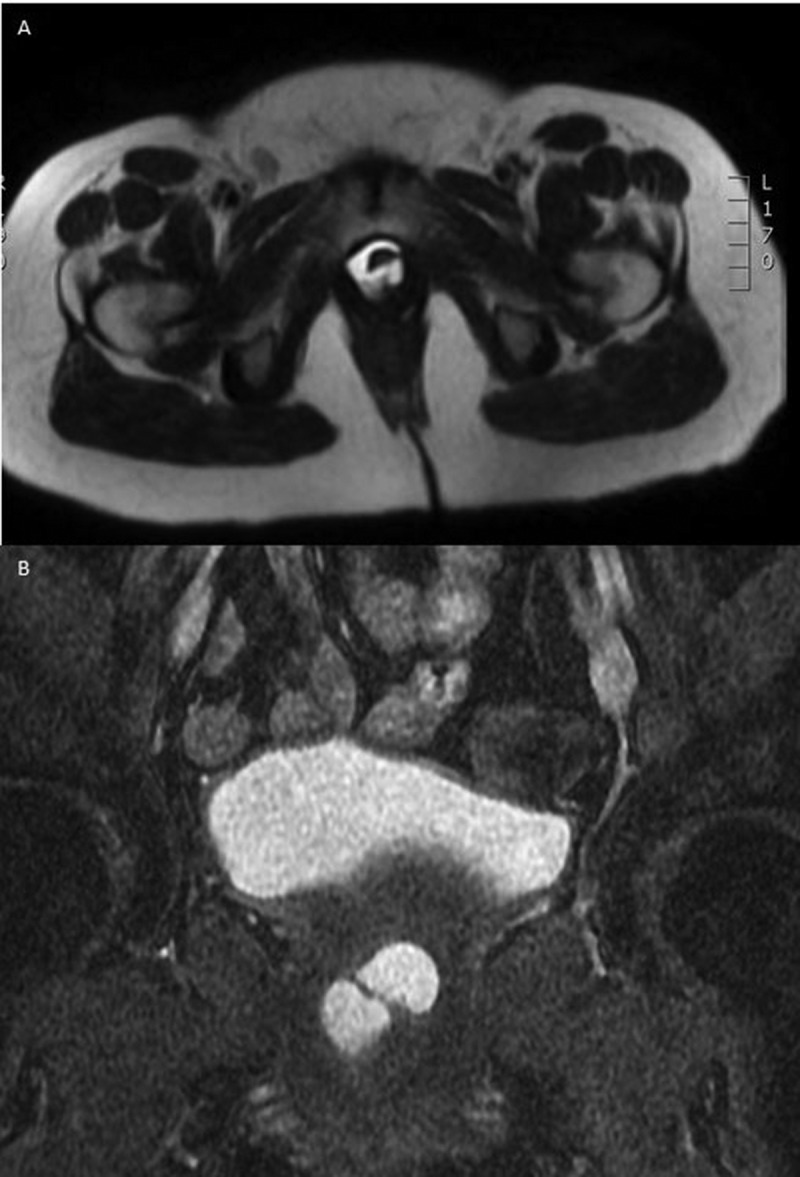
(a) Axial T2-weighted MRI image of a 3.7-cm circumferential UD. (b) Coronal T2-weighted MRI image of the same 3.7-cm circumferential UD.
